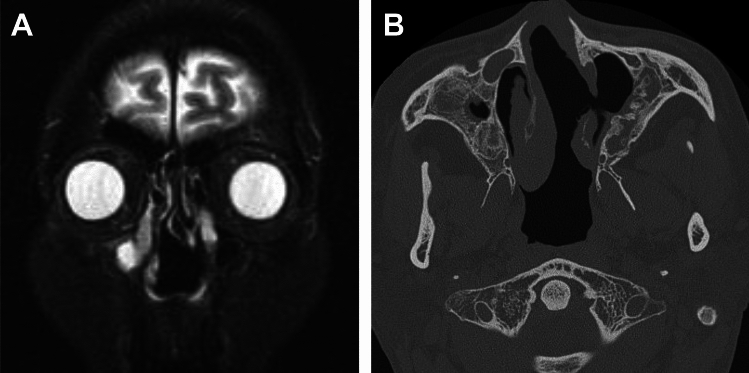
Sinonasal involvement in a 50-year-old man with granulomatosis with polyangiitis. (A) Coronal STIR shows septal defect and maxillary sinus hyperintensity. (B) CT shows destruction of the nasal septum and left inferior concha, as well as neoosteogenesis of the maxillary sinuses
